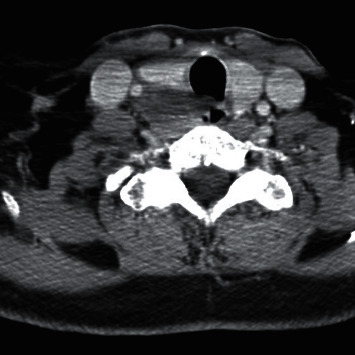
First CT scan of the neck after the initiation of pembrolizumab, 4 doses (axial image).
